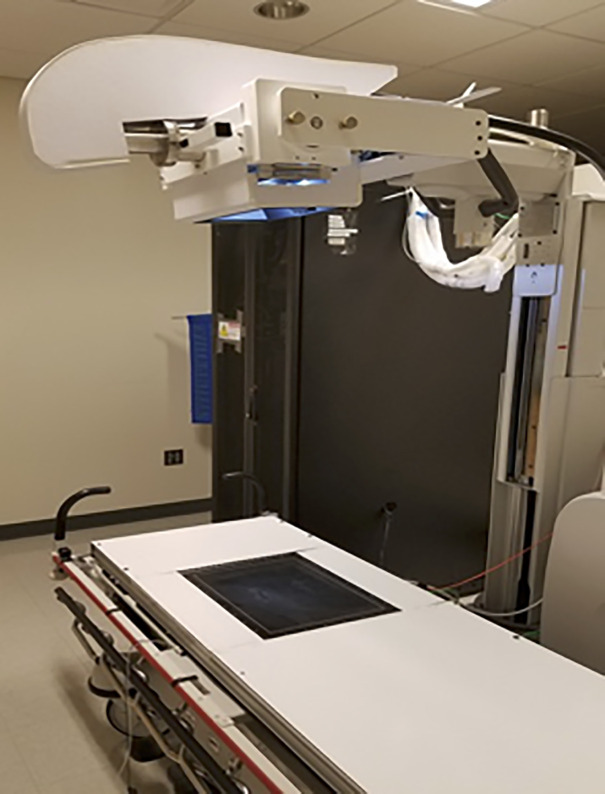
Stationary Digital Chest tomosynthesis system, CNT array retrofitted into a portable X-ray system.
